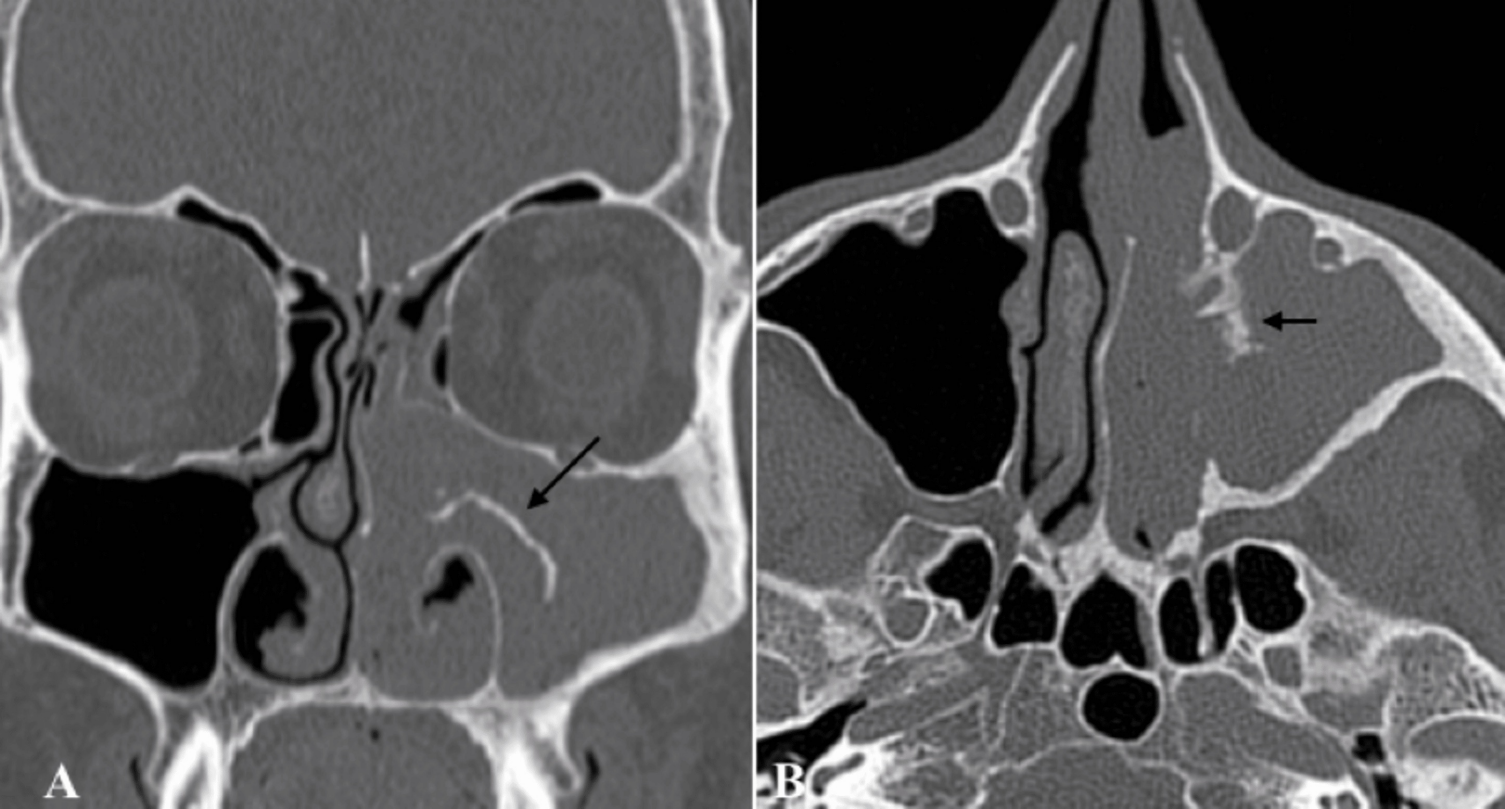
Maxilla medial wall IP CT sections (coronal and axial, respectively) showing an IP, indicated with an arrow, originating from the medial wall of the left maxilla CT: computed tomography; IP: inverted papilloma
